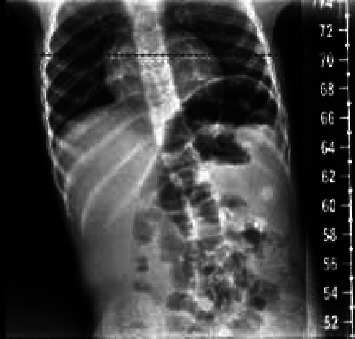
Scoliosis found on X-ray.
